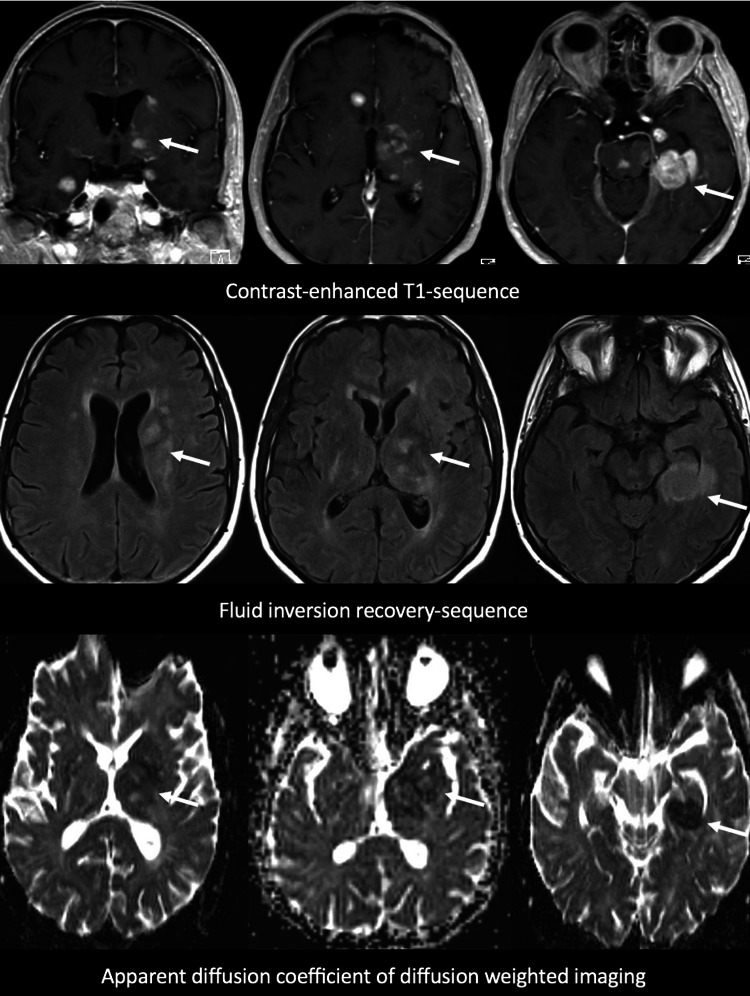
Example of a patient with CNS lymphoma with multiple lesions (mCNSL). Top: contrast-enhanced T1 sequence, showing periventricular tumor growth affecting the basal ganglia, brain stem, and the left hippocampus. Middle: fluid inversion recovery (FLAIR) sequence demonstrating the signal of tumor lesions and perilesional edema. Bottom: diffusion weighted imaging (DWI) with the apparent diffusion coefficient (ADC) indicating a diffusion restriction of the lesions
